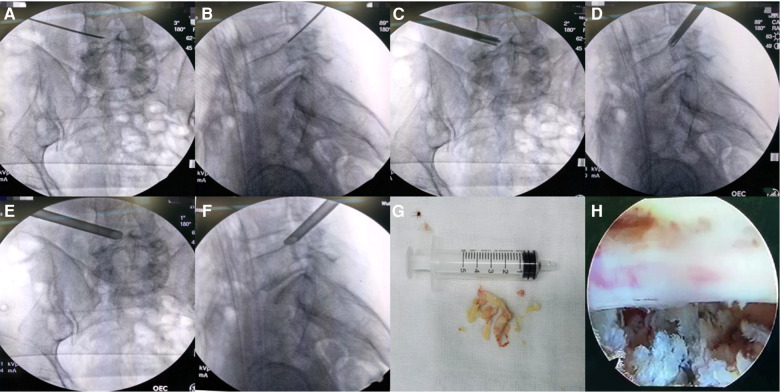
(A,B) anteroposterior and lateral position x-ray radiographs indicate the location of the spinal needle; (C,D) anteroposterior and lateral position x-ray radiographs indicate the location of the serrated reamer, which was used for zygapophyseal plasty; (E,F) anteroposterior and lateral position x-ray radiographs indicate the location of the working channel; (G) extracted the prolapse of nucleus pulposus; (H) sufficient decompression of the nerve root was seen under the endoscope.
